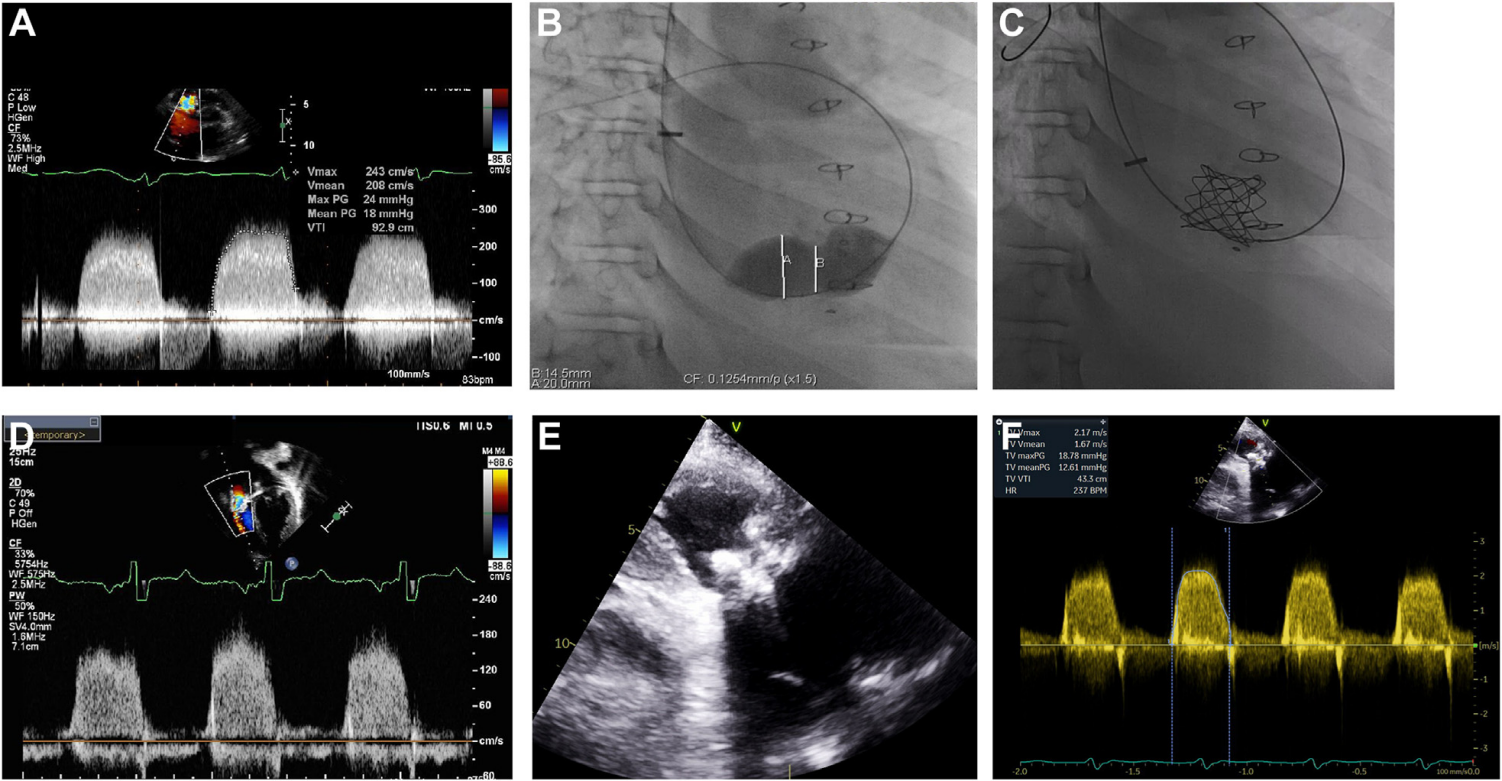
(A) Severe stenosis of bioprosthetic tricuspid valve. (B) Balloon angioplasty of bioprosthetic tricuspid valve. (C) Placement of Melody valve in tricuspid position. (D) Melody valve inflow Doppler at 5 years. (E) Melody valve in 2D at 11 years. (F) Melody valve inflow Doppler at 11 years.
